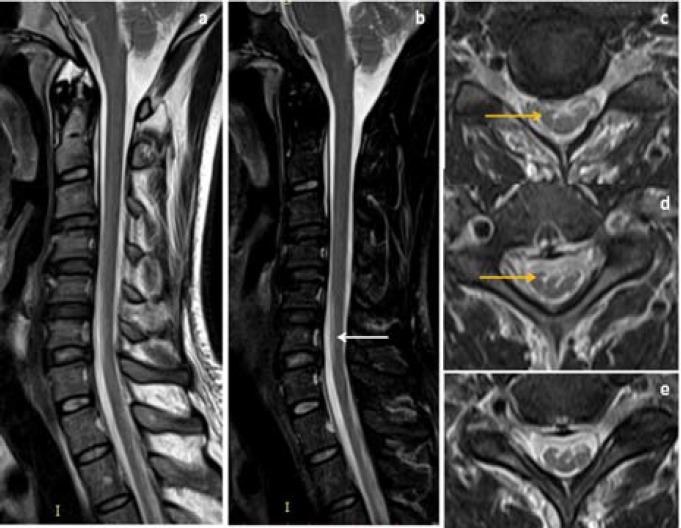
In a 18-years old man neutral MRI sagittal T2W and fat suppressed T2WI (a, b) showed lower cervical cord atrophy with hyperintensities in anterior cervical cord extending from C5 to C7 vertebral level, axial T2WI (c, d and e) showed asymmetrical hyperintensities in AHC (yellow arrows) and anterior cervical cord
